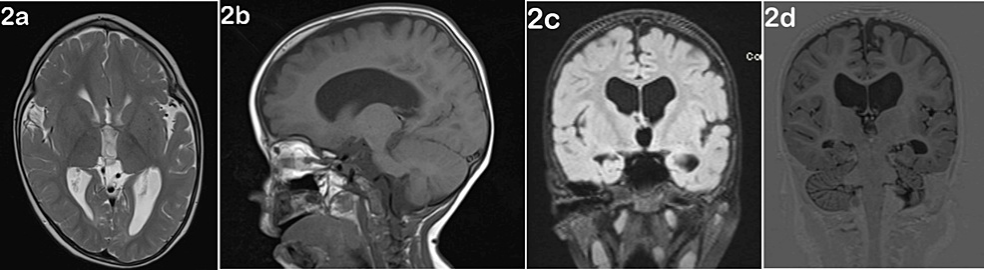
Cerebral MRIs Cerebral MRIs indicate the pathognomonic aspect of "boat-shaped" skull, with a narrow and long cranial shape and a metopic ridge: (a) axial T2; (b) sagital T1; (c) coronal FLAIR; (d) coronal T1 SPACE.
